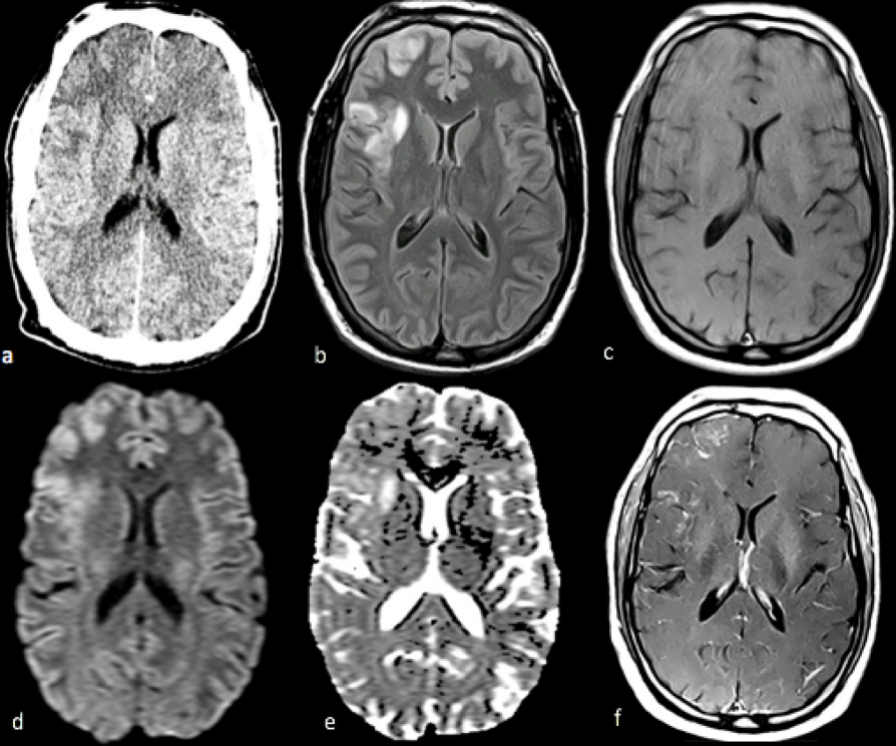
Head NCCT (a) and MRI (b – f) showing hypodensity and mild mass effect on NCCT (a), high signal on FLAIR (b) and patchy *T*1W gadolinium enhancement (c, f) in the cortex and subcortical white matter of the right anterior opercular frontal and insular regions, with additional involvement of the ipsilateral anterior frontal convexity, without restriction on DWI/ADC (d, e). ADC, apparent diffusion coefficient; DWI, diffusion-weighted imaging; FLAIR, fluid-attenuated inversion-recovery; NCCT, noncontrast head CT.
